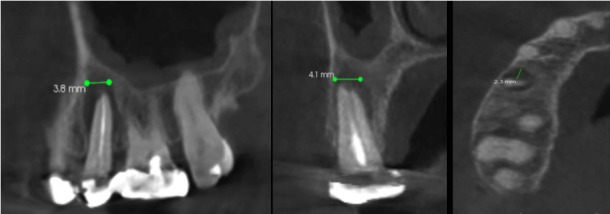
Measurement of the size of periapical lesion on cone-beam computed tomography (CBCT) image. The arrows show (A) Mesiodistal measurement on sagittal section. (B) Buccolingual measurement on coronal section of CBCT. (C) Measurement of depth of periapical lesion on axial section.
